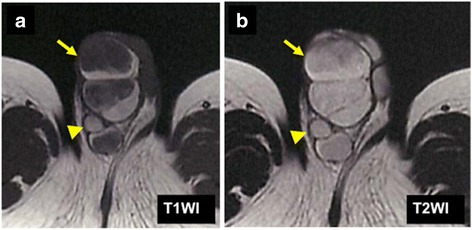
The MRI findings. a T1-weighted image showed the mosaic pattern (e.g., T1-low lesions and T1-high lesions were co-existed). b All of the tumor revealed the high intensity on T2-weighted image. (*Arrow heads* indicate right testis.)
